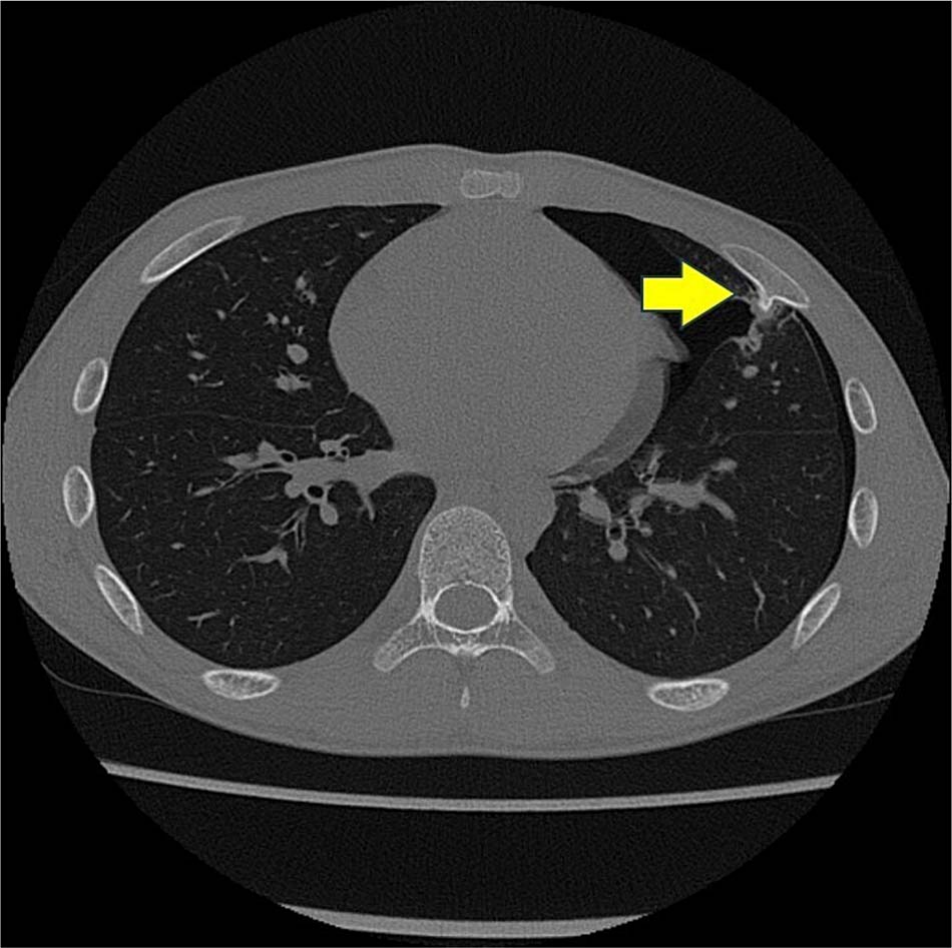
Chest computed tomography scan showing a left pneumothorax and a mass arising from the fifth rib to the thoracic cavity (arrow). The cortical bone and bone marrow continue to move from the fifth rib.
